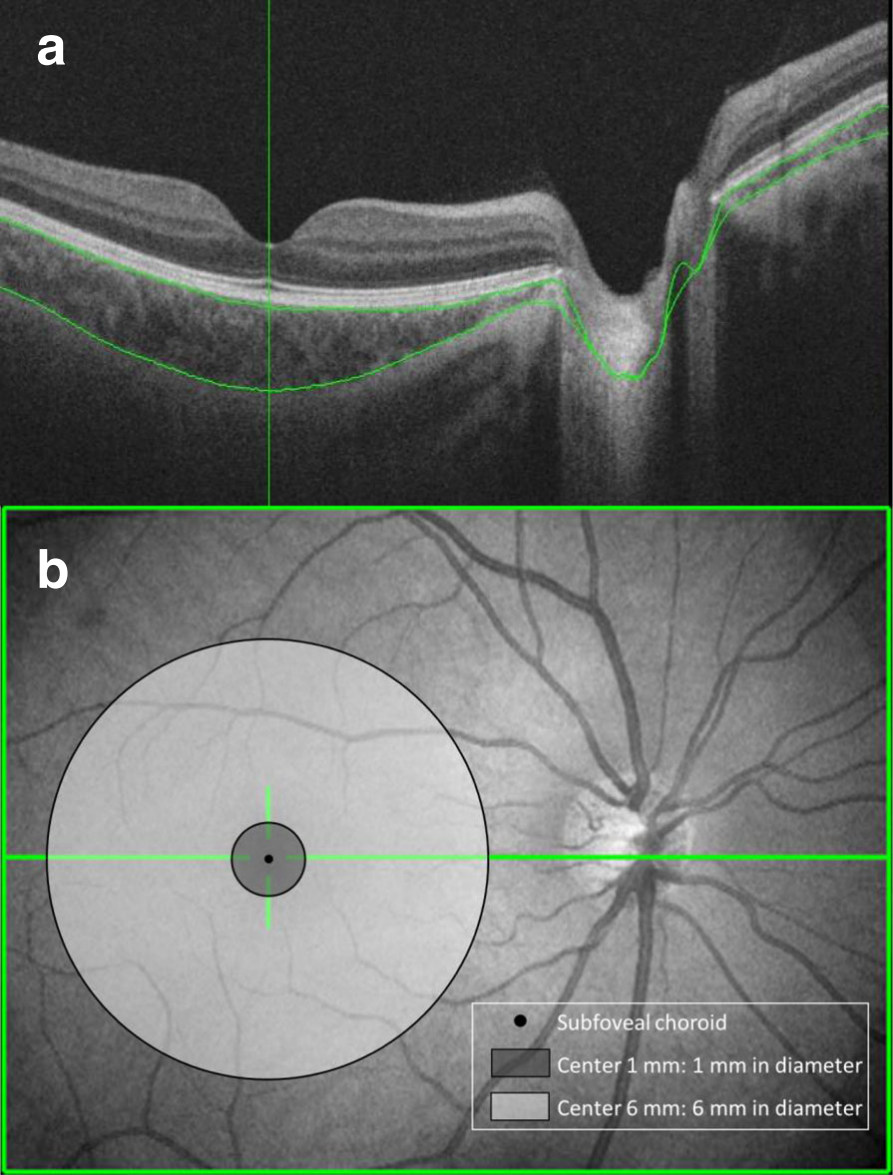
Measurement of choroidal thickness using swept-source optical coherence tomography. a The scanning protocol used was the 3D macula with a scan density of 512 × 256 covering a 12 × 9 mm^2^ area. The choroidal thickness was automatically analyzed using built-in tools. b The three defined macular regions were: subfoveal choroidal thickness (SFCT), radii of 0.5 mm choroidal thickness (center 1 mm CT), and radii of 3.0 mm choroidal thickness (center 6 mm CT)
